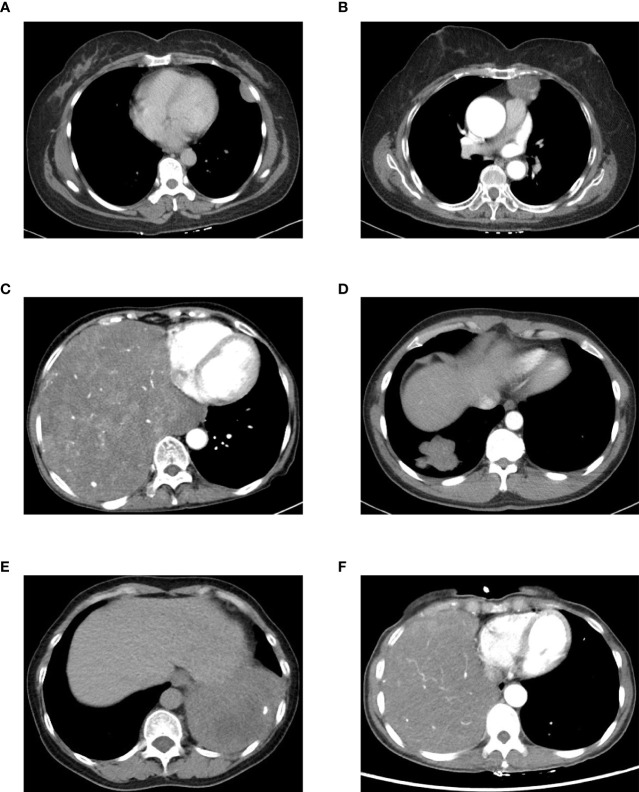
CT features of the 50 SFTC patients: (A) a mound-like lesion with uniform internal density in the left pleura; (B) a circular lesion in the left mediastinum with vascular shadows inside; (C) a huge lesion in the right thoracic cavity with uneven internal density, showing uneven enhancement; (D) a lobular lesion in the right lung with burr signs; (E) a lesion with calcification and necrosis in the left thoracic cavity; and (F) a lesion with multiple tortuous vascular shadows in the right thoracic cavity.
